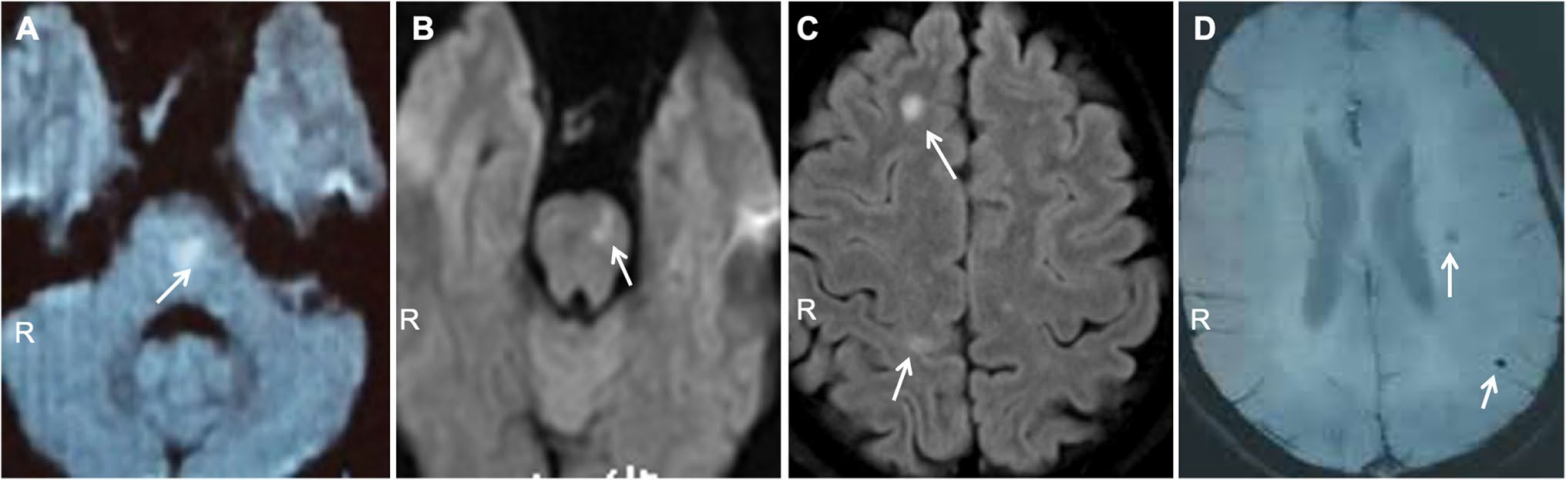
Subsequent MRI brain of the index case (III10). DWI showed an acute infarct in the left paramedian pons (a and b) and in the subcortical white matter of right frontal lobe and parietal lobe (c). Intracerebral microhemorrhages (d) were shown on the susceptibility-weighted imaging (SWI)
